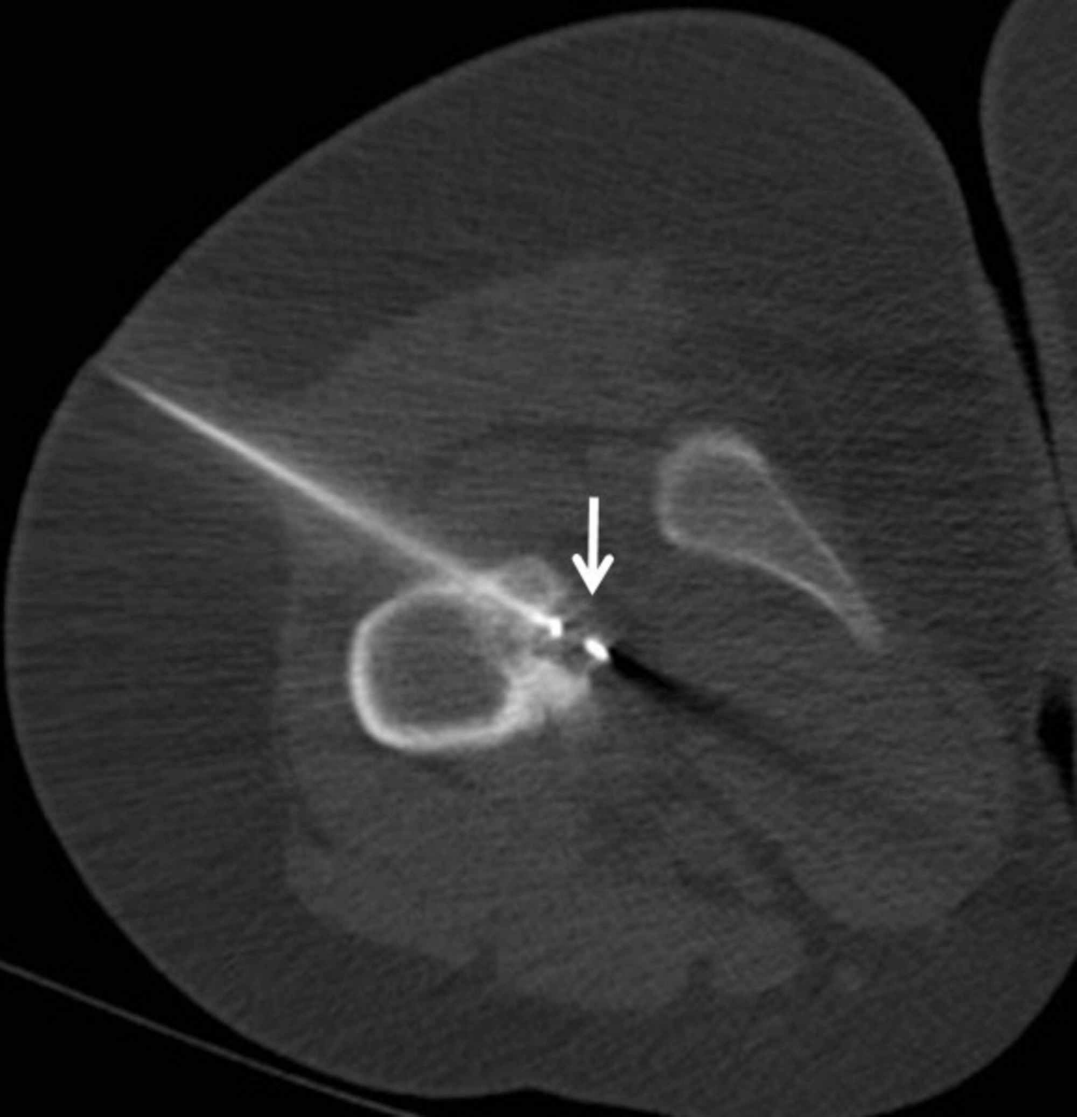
Axial CT image shows CT-guided placement of a cryotherapy probe. A 16-year-old female with lytic lesion of the right femoral lesser trochanter cortex. Axial CT shows CT-guided placement of a cryotherapy probe into the lesion from posterolateral approach.
